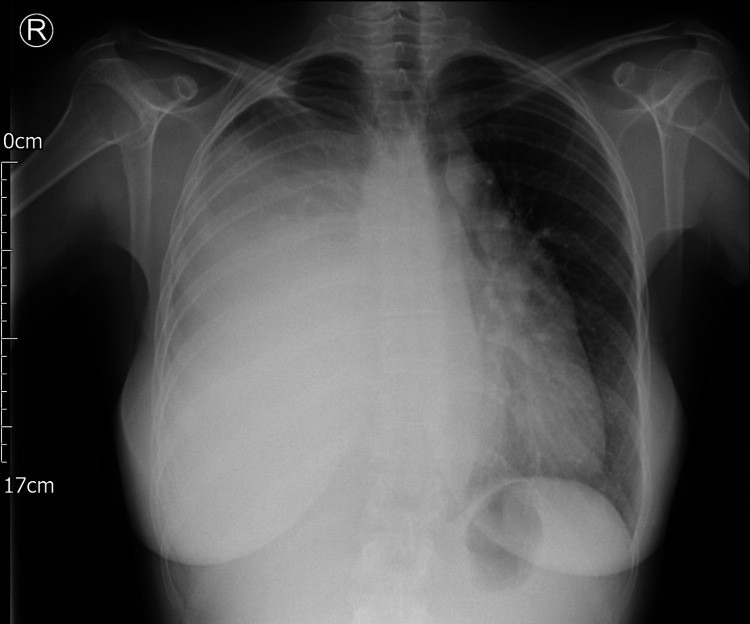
A posteroanterior (PA) chest X-ray showing a near-complete opacification of the right hemithorax with displacement of the mediastinum towards the left.
